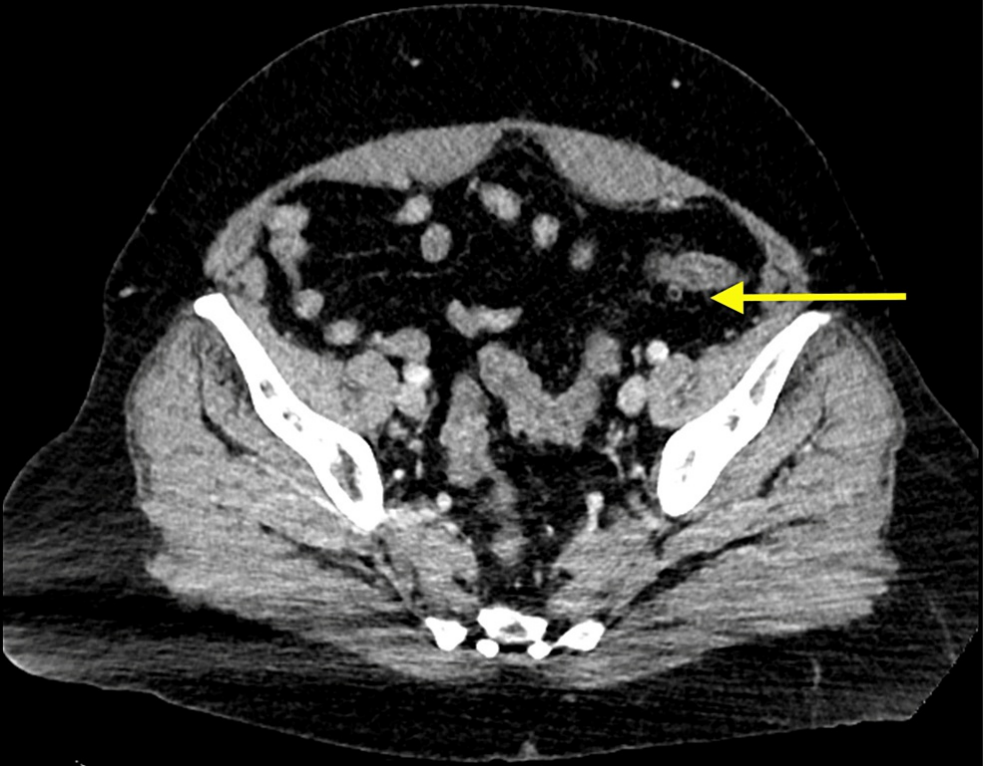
CT scan showed thickened colonic wall with mild fat stranding (yellow arrow)
